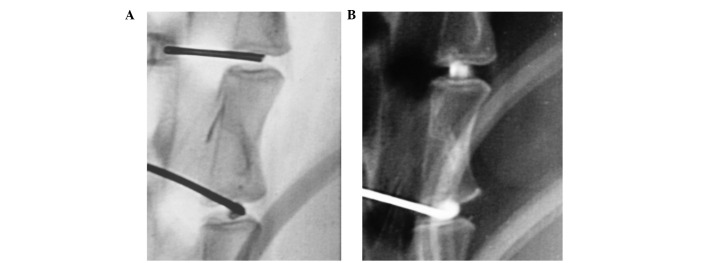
Lateral view of the X-ray film prior to and following cement injection.
